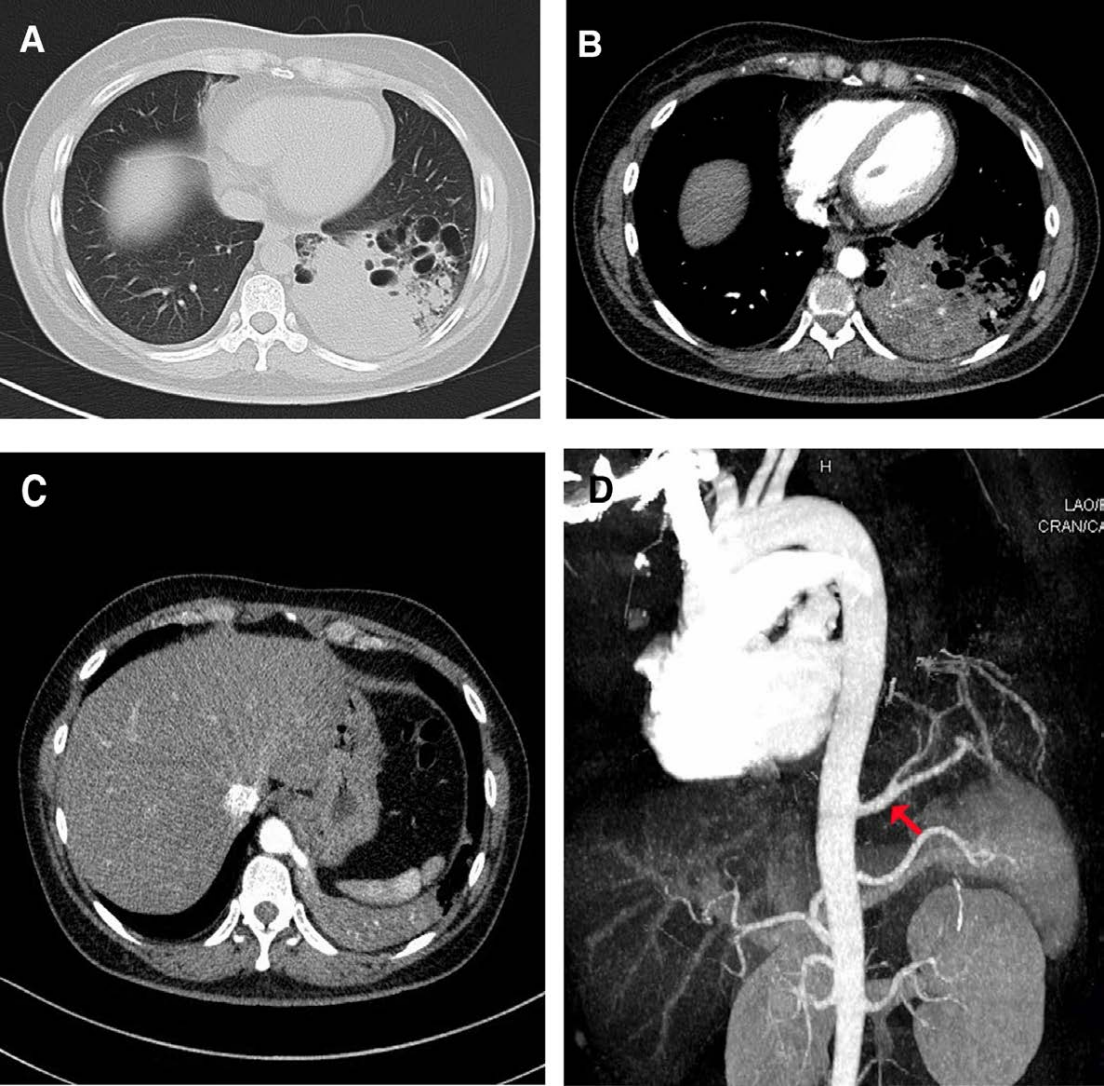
(A and B) Chest contrast-enhanced CT scan of the thorax showed that the left lower lobe had been very extensively replaced by multi-cystic changes and diffuse consolidation of the inflammatory. (C and D) CT scanning 3-dimensional reconstruction confirmed the diagnosis of left lower lobe pulmonary sequestration by clearly demonstrating the aberrant feeding vessels (red arrows).
